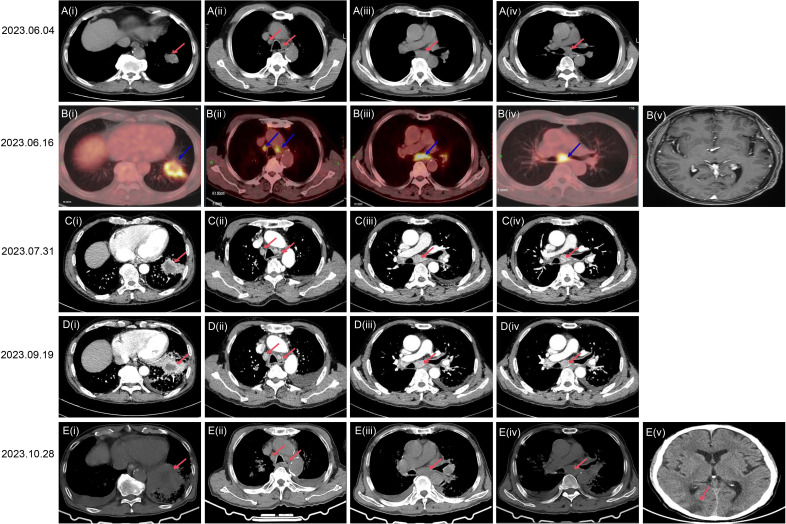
CT, PET and MRI scan images showing various scans of the chest and brain during different treatment time.
